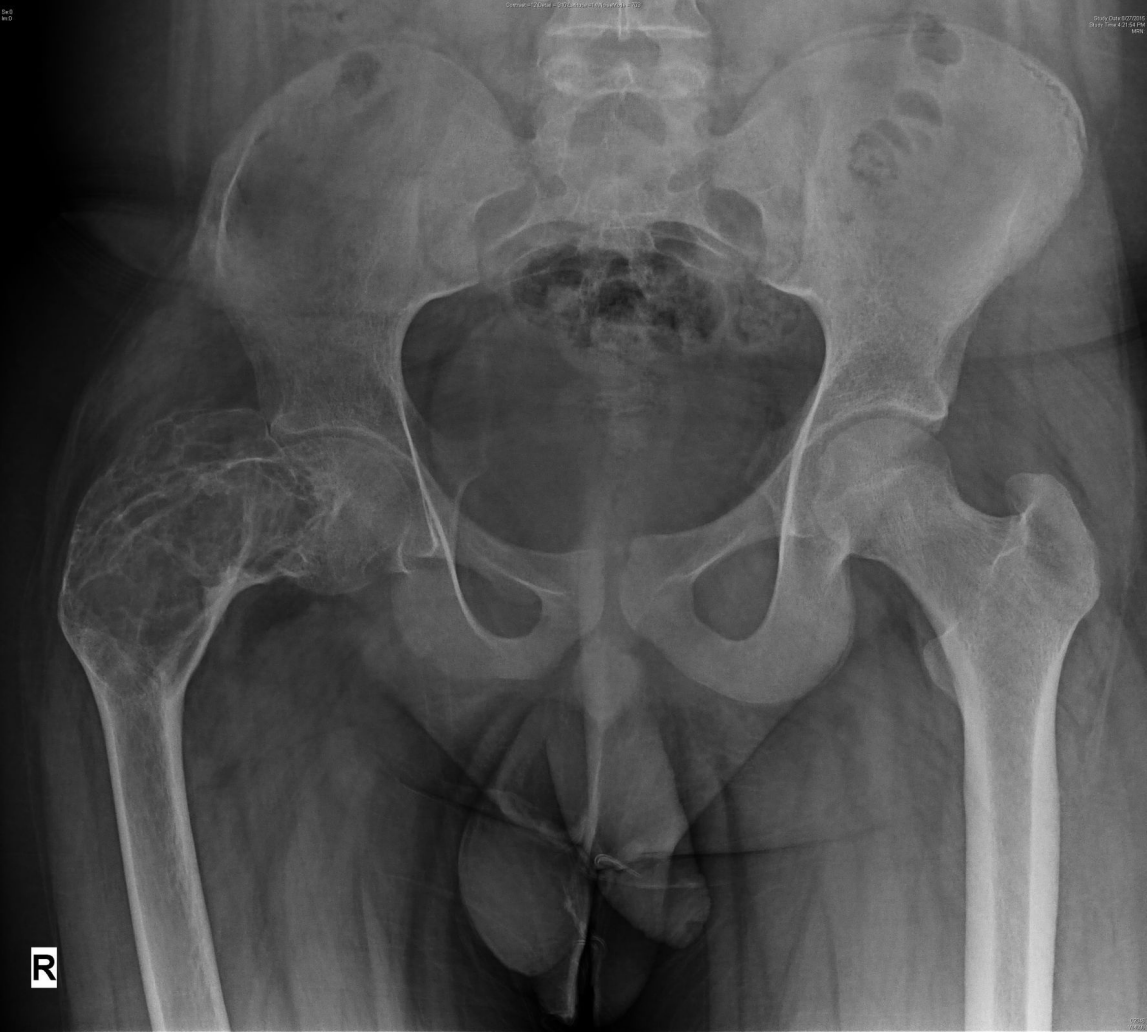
Anteroposterior X-rays of the pelvis with both hips showing large, well-defined, expansile lytic lesion in the metaphysis of the proximal femur with a breach in the lateral cortex
